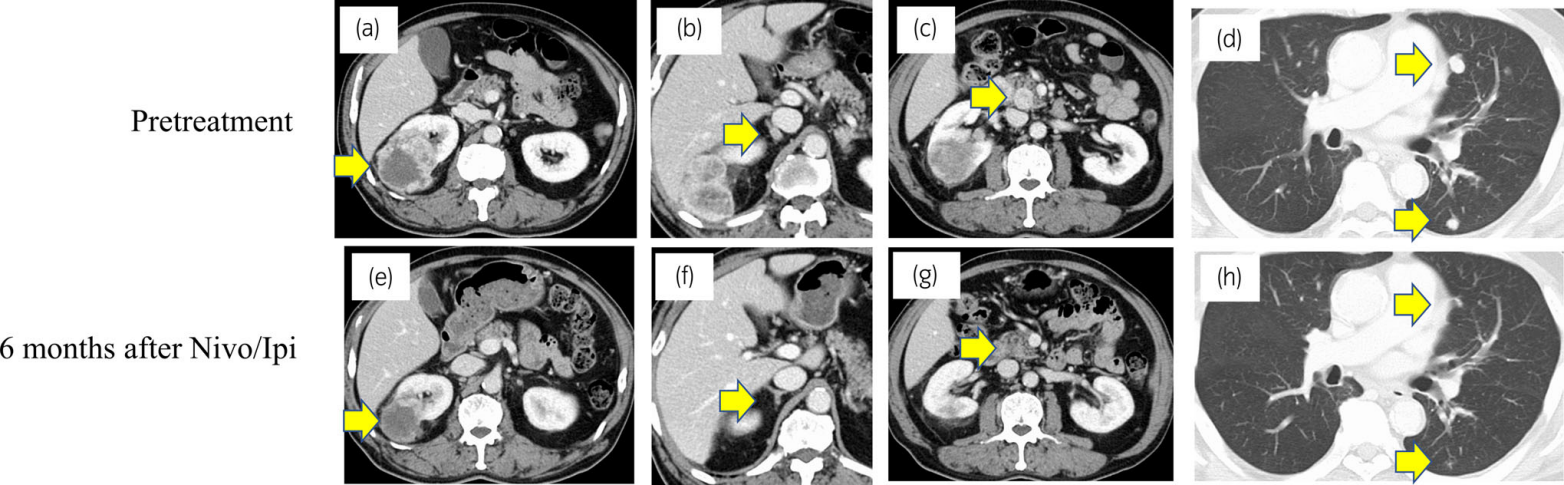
Computed tomography scan findings before treatment and 6 months after nivolumab plus ipilimumab treatment in primary site (a, e), right adrenal (b, f), pancreas (c, g), and lung metastases (d, h).
